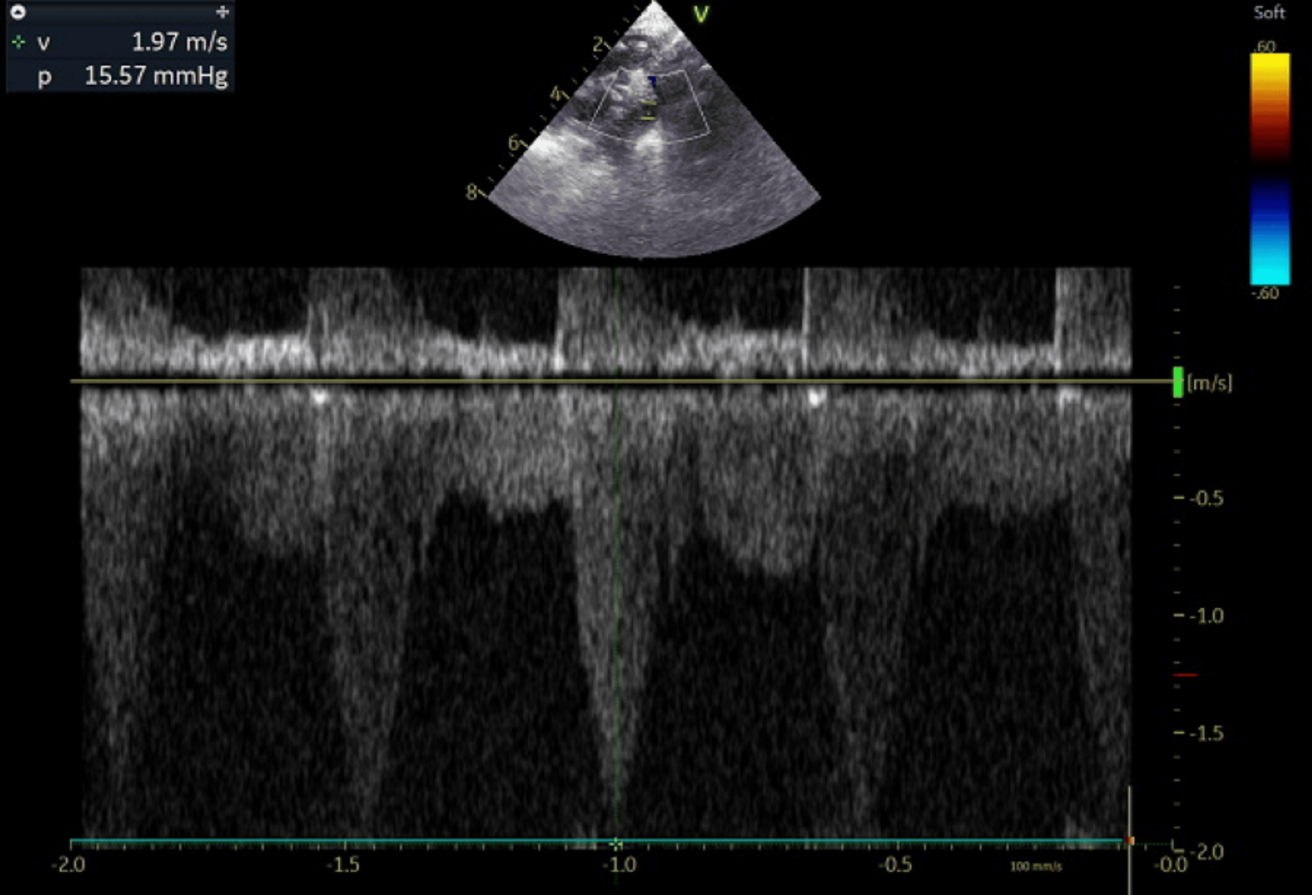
Echocardiography (Eco 2D/ Doppler) in the first hours of life (Case 2): "gothic" aortic arch with flow acceleration at the isthmus with a gradient of 15 mmHg.
